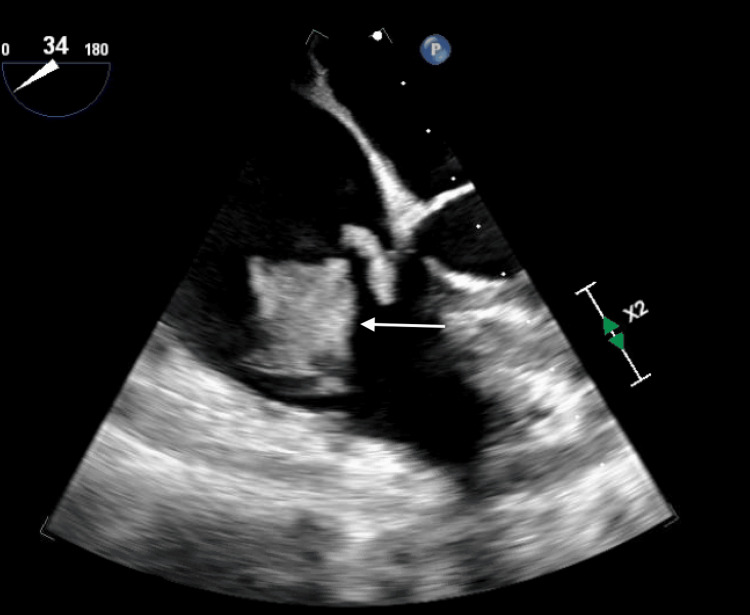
TEE showing a large mass on the tricuspid valve. The white arrow shows mass on the tricuspid valve. TEE, transesophageal echocardiogram
